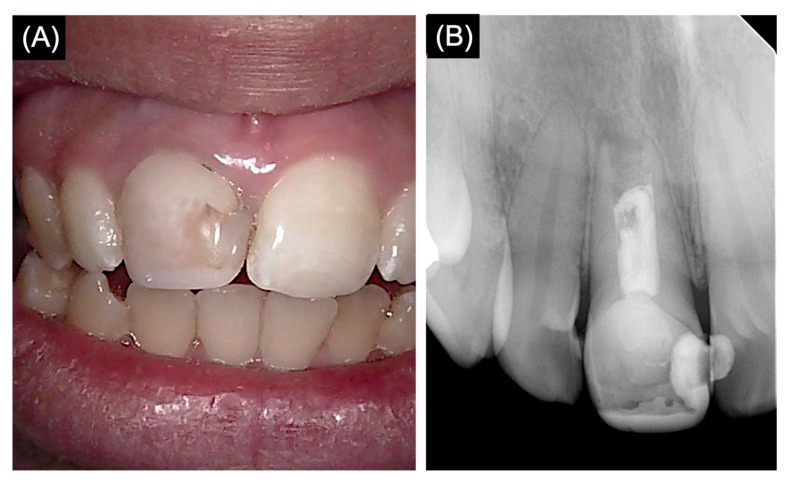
(A) Clinical photograph of tooth number 11. (B) Preoperative periapical radiograph of tooth number 11.
